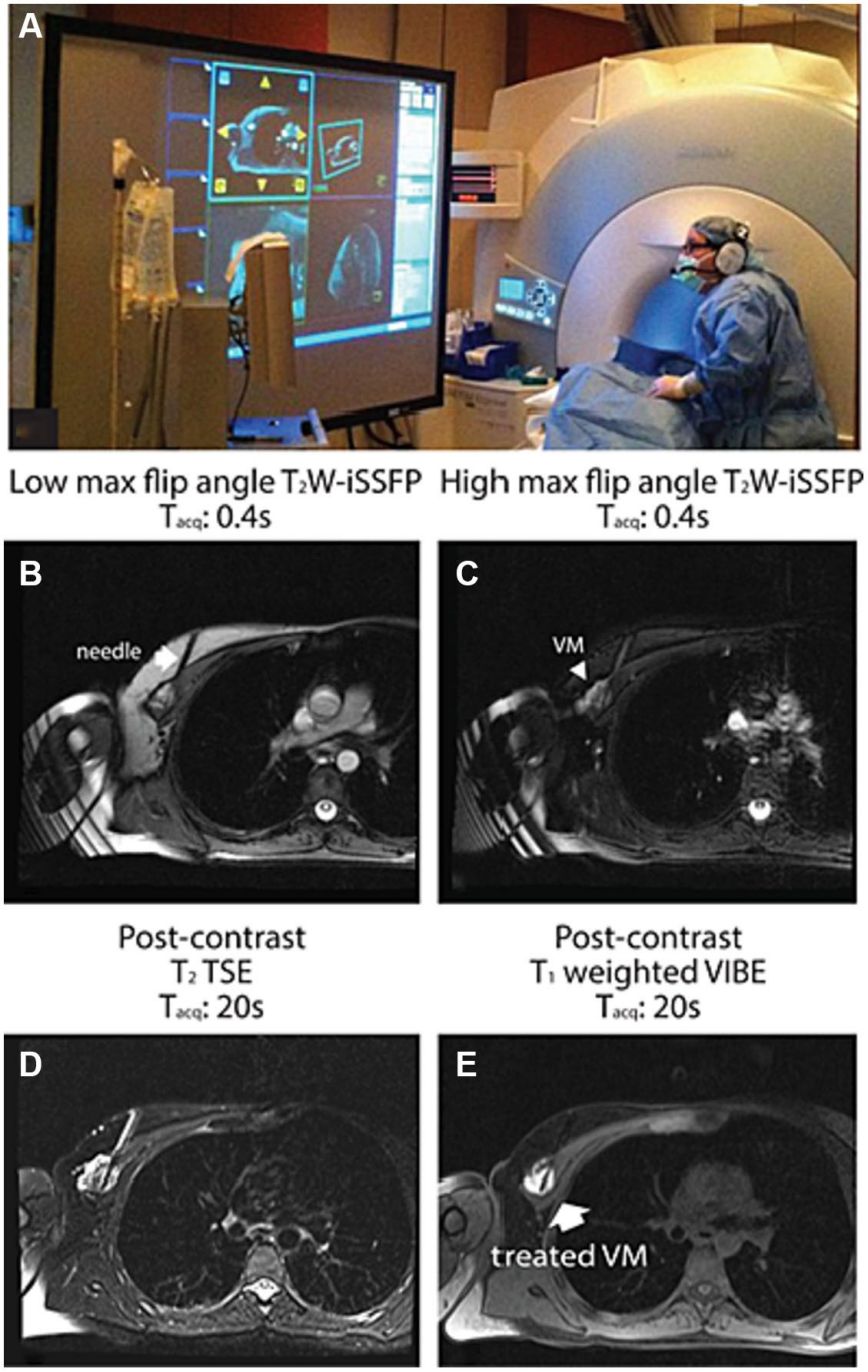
An example of a MR-guided sclerotherapy using T2W-iSSFP. This patient underwent treatment for a deep chest wall VM which was not accessible by US guidance. (A) Real-time MR video was integrated into an interventional guidance visualization software and displayed on an in-room projector. (B) High and (C) low max flip angle real-time T2W-iSSFP images sampled during needle insertion. Real-time T2W-iSSFP offers heavy T2-weighting and high image quality to visualize the lesion and its extent, as well as the surrounding critical structures such as the normal blood vessels and the lung. Low max flip angle T2W-iSSFP emphasizes the depiction of the needle [the arrow in (B)] and the surrounding soft tissue. High max flip angle T2W-iSSFP provides heavily T2-weighting for visualizing the VM emphatically [arrowhead in (C)]. (D) Post-contrast T2-TSE and (E) post-contrast T1-weighted VIBE 20 demonstrate the success of the needle placement and the filling of the sclerosant (sodium tetradecyl sulfate). Reproduced with permission, John Wiley and Sons, Inc. (42).
